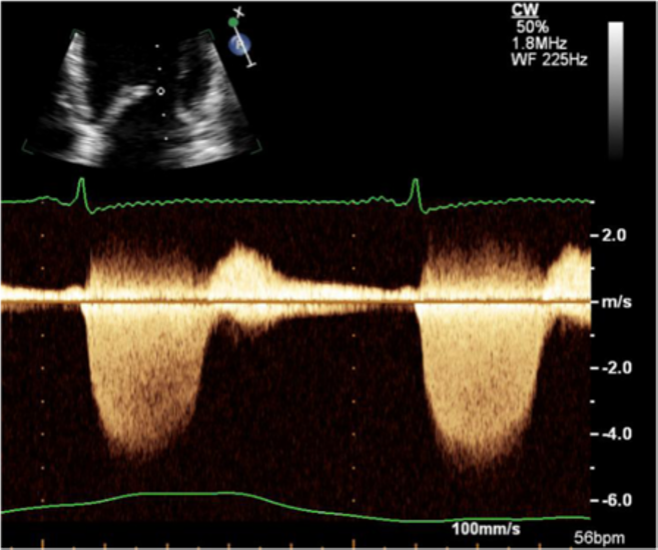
Dense Doppler Signature in Severe MR. This continuous wave Doppler interrogation of an MR jet taken from the apical four chamber view shows a very dense Doppler profile, which is consistent with severe MR. The peak MR velocity is 4.5 m/s, and this value would be used in the denominator of the calculation of EROA.
